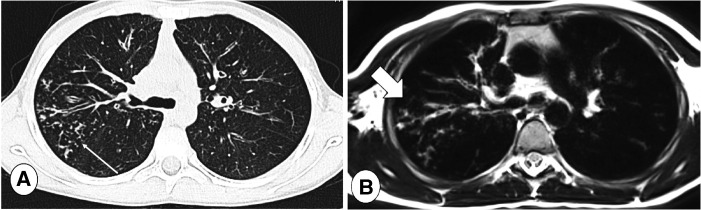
Axial CT (A), lung window, and axial T2-weighted MR image (B) in a CF patient with tree in bud in the posterior segment of the right upper lobe (thin arrow). Note loss in definition of lung abnormalities in the MR image, where peripheral abnormalities may be not be visible in the MRI sequence (B, thick arrow).
